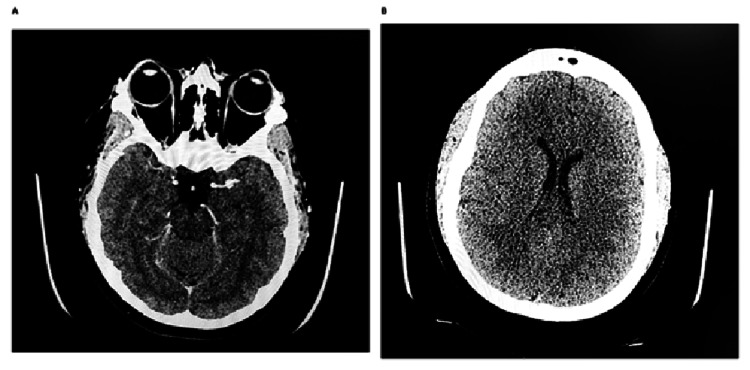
CT venography and CT of the head Neuroimaging during admission. (A) CT venography demonstrating no evidence of cerebral venous sinus thrombosis. (B) Axial CT of the head demonstrating no acute intracranial abnormality, mass effect, or extra-axial collection. CT: computed tomography
